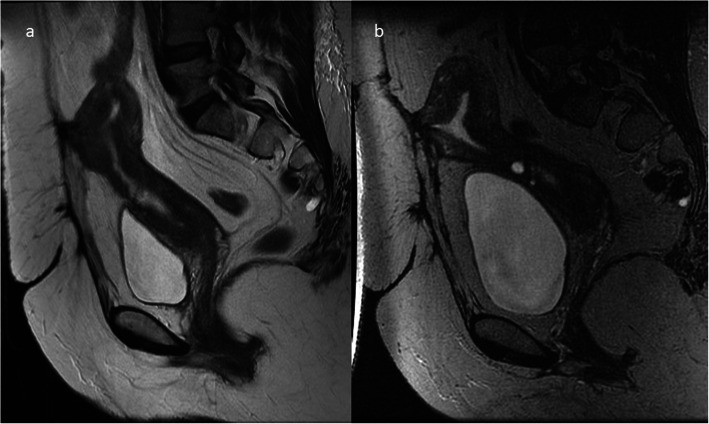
Pelvic MRI in the sagittal plane. FSE/T2WI (a). CUBE/3D/T2WI (b). Thick hypointense adhesions between the abnormally flexed uterus and an anterior abdominal wall
